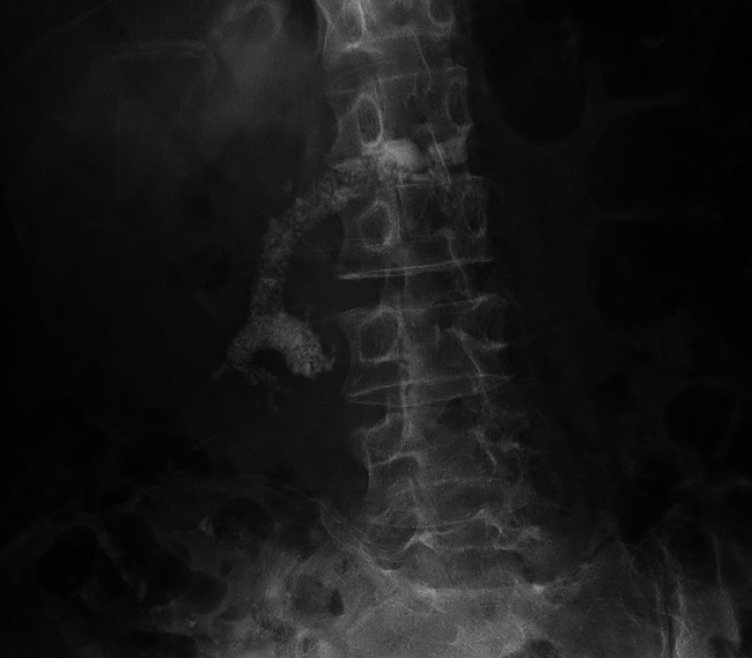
Radiographic image showing the appearance after three sessions of extracorporeal shock wave lithotripsy.
